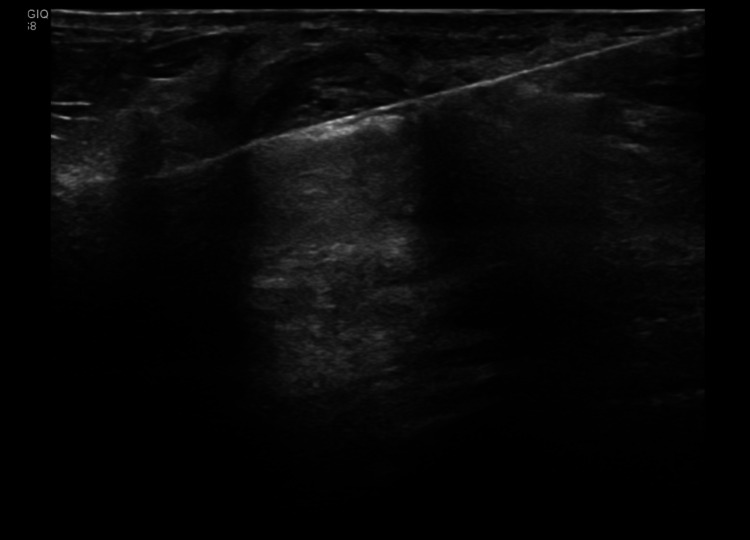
Ultrasound image of the palpable mass during diagnostic core biopsy Ultrasound image demonstrating the biopsy needle, 14G core biopsy samples were taken with three passes from medio-lateral approach.
